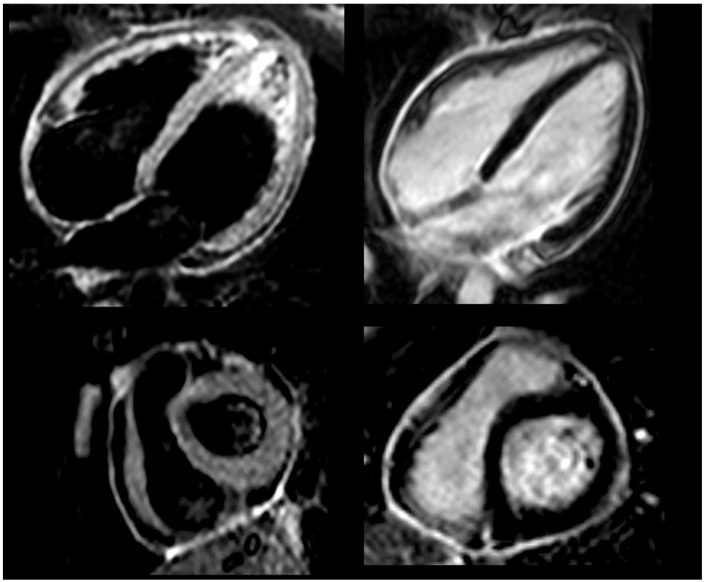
Cardiac magnetic resonance before anti-IL-1 treatment, showing bright signal in T2-weighted black blood STIR sequences (left panel) and intense delayed pericardial enhancement (right panel).
